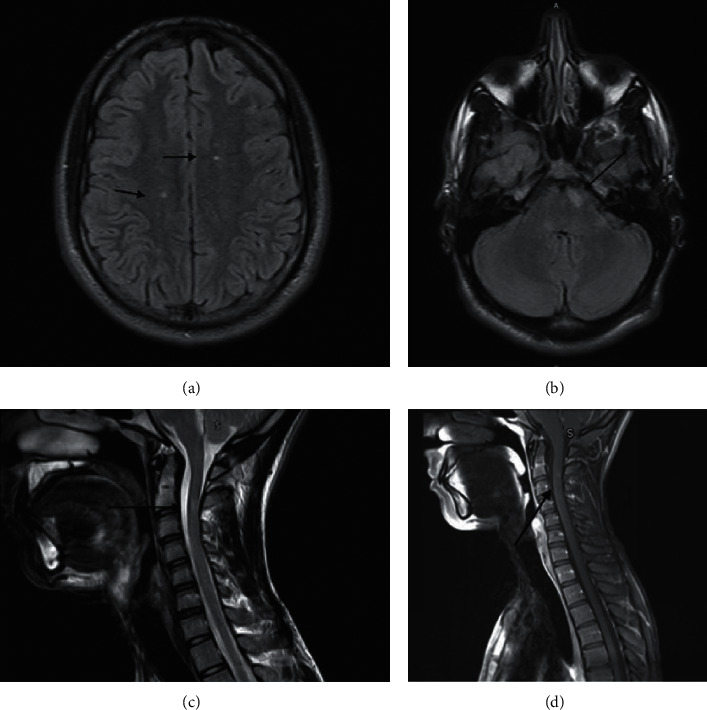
(a) There are few small foci of T2 and FLAIR hyperintensity noted in the periventricular white matter (bilaterally), perpendicular to the ventricular margin. (b) There is a small area of T2 and FLAIR hyperintensity noted in the left paramedian anterior pons. ((c), (d)) There is a small area of T2 hyperintensity with corresponding postcontrast enhancement noted in the cervical spinal cord at the C2-C3 level.
